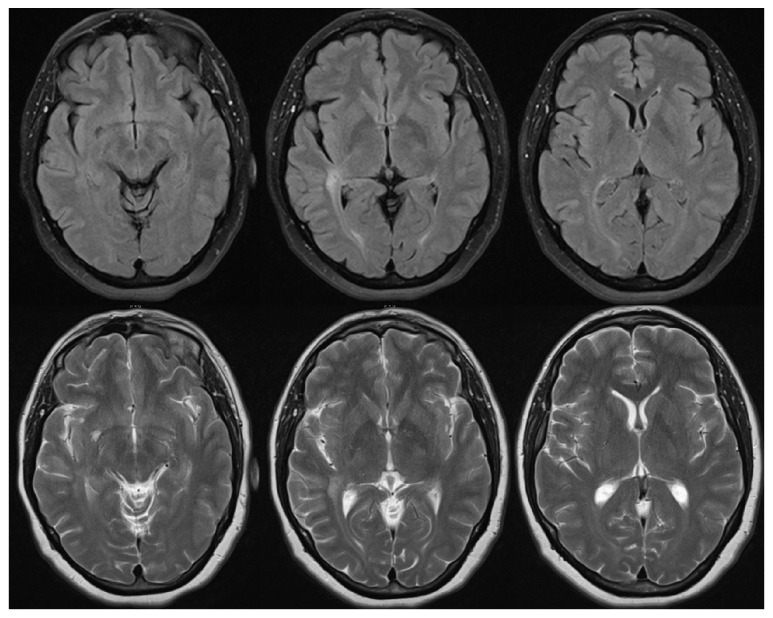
MRI of the brain showing almost complete resolution of the previously noted changes.
